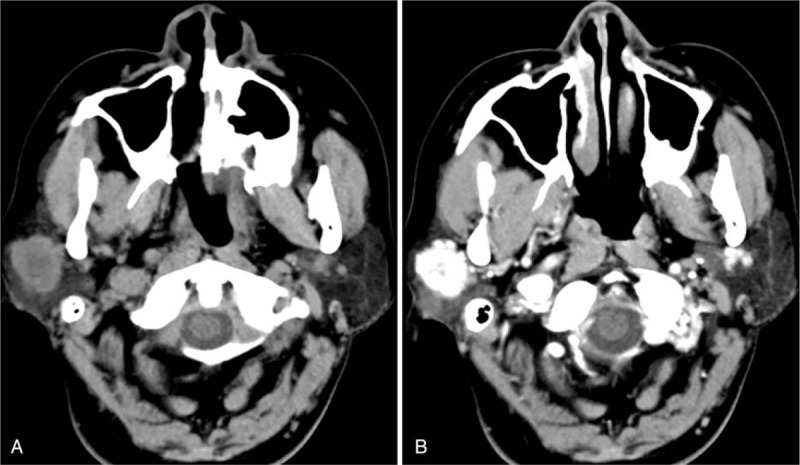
A 48-yr-old woman with myopericytoma in the right parotid gland. (A) Pre-contrast CT image revealing a 2.2 cm × 1.4 cm, relatively well defined, heterogeneous iso-density mass with central lower density in the right parotid gland; (B) Enhanced scan revealing mass with significantly heterogeneous enhancement and central irregular non-enhanced area. The CT values of the significant-enhanced areas in pre-contrast and enhanced scans were 44.82 HU and 157.32 HU, respectively.
